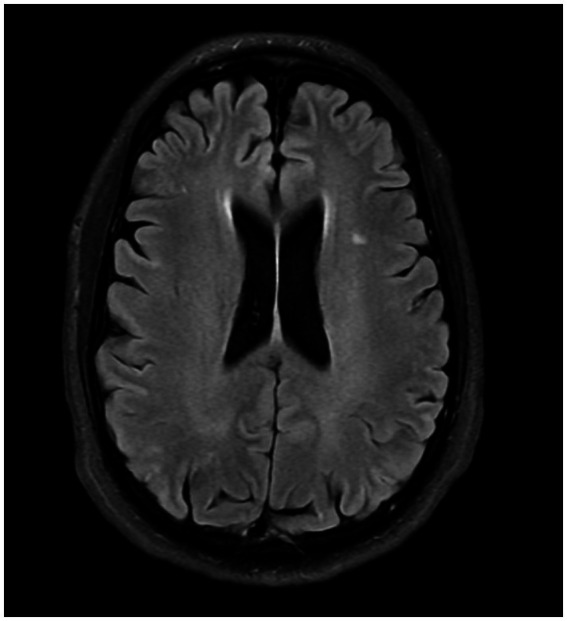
Subclinical brain infarct, FLAIR sequences of MRI.
